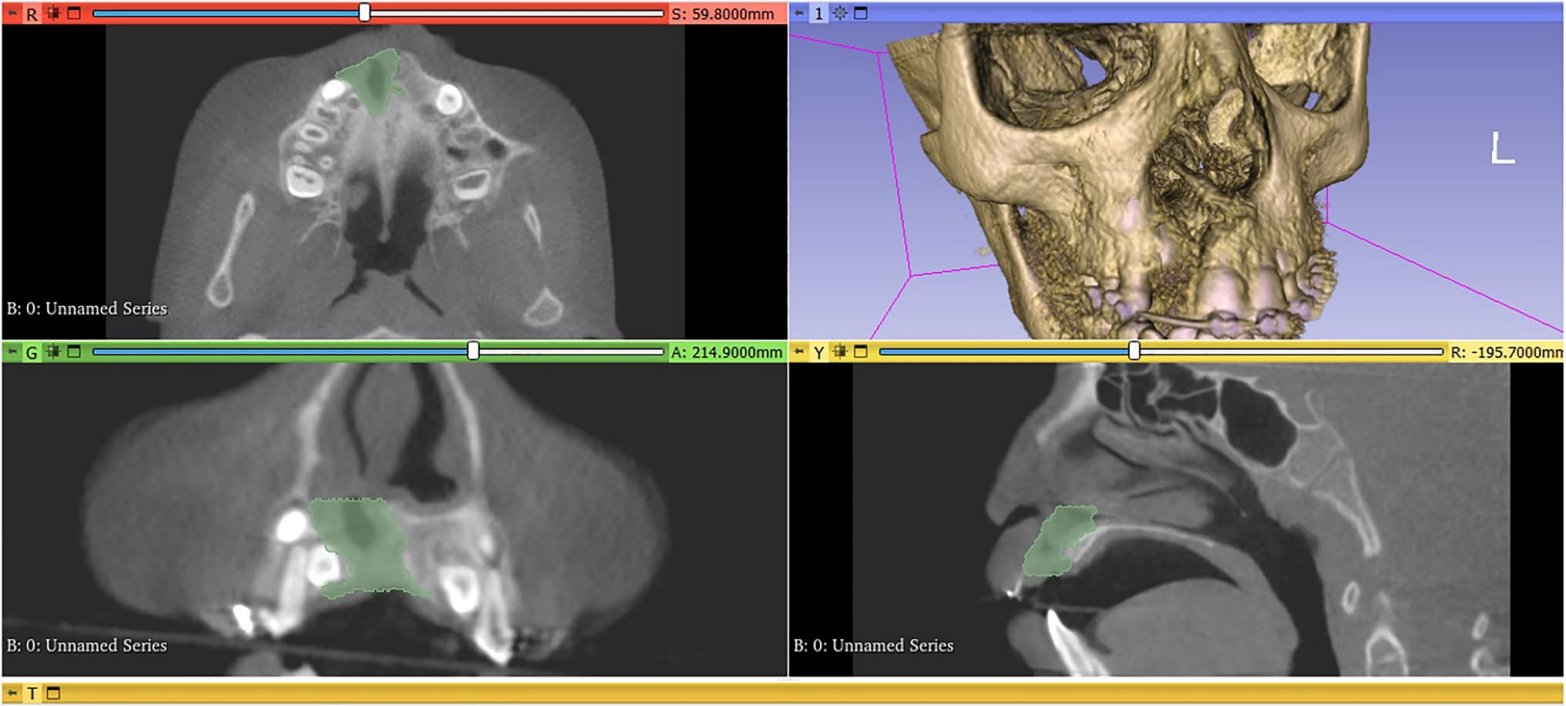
Radiographic photo showing manual segmentation of the cleft area using the (segment statistics) module in 3D Slicer for presurgical cleft volume determination
